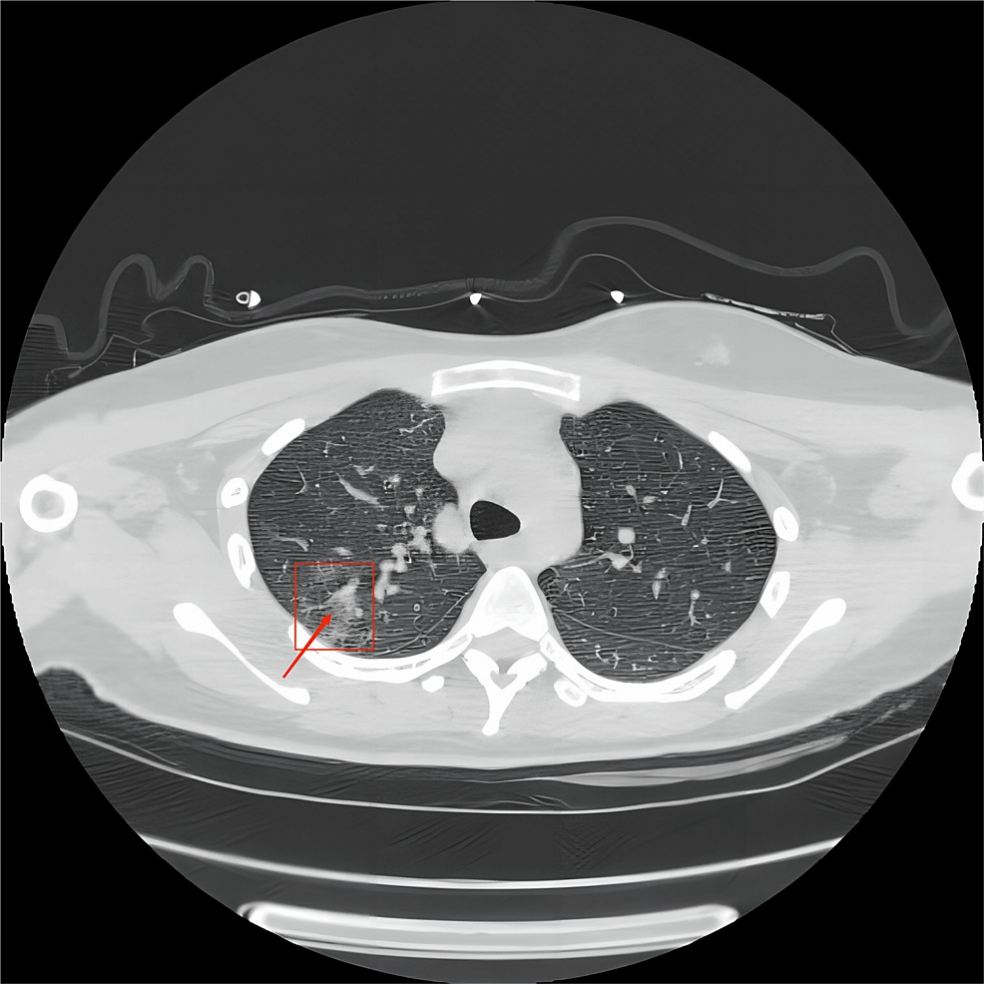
Chest computed tomography scan indicating exudative changes in the right lung with possible inflammation.
